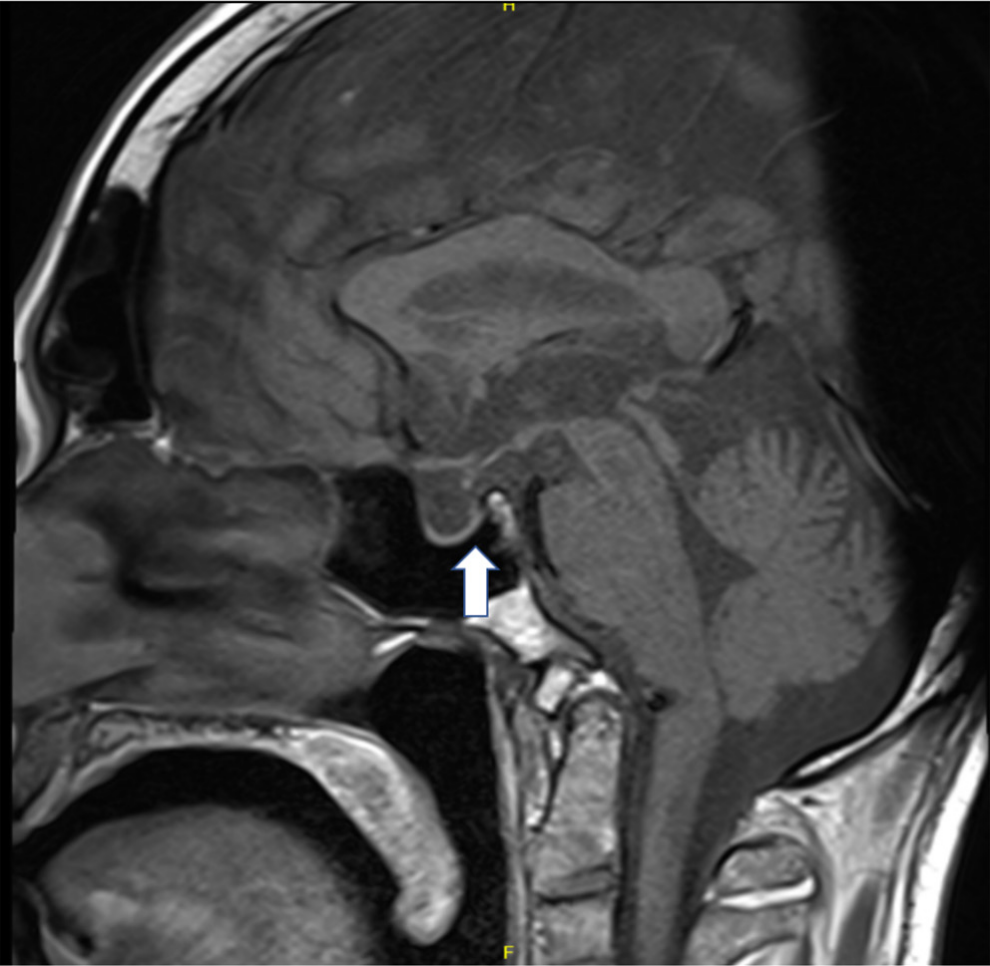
Sagittal T1-weighted MRI imaging with empty sella appearance, with absent posterior pituitary bright signal labelled with arrow.
